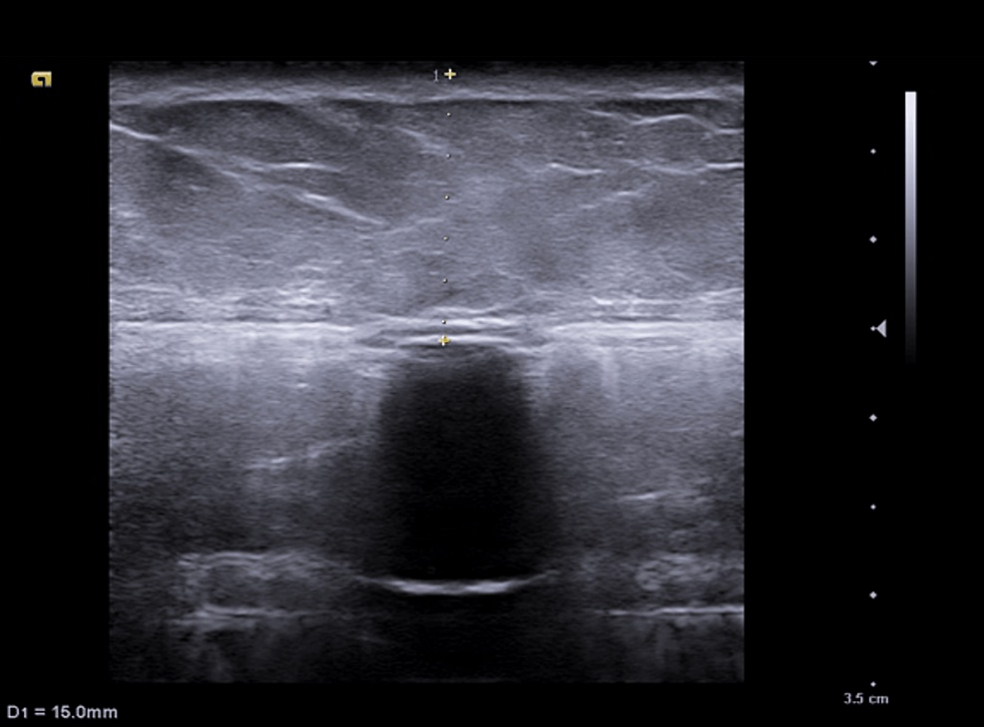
Ultrasound imaging of ITB pump. The surface of the intrathecal pump is identified by a hyperechoic line with acoustic shadowing. A hypoechoic shadow represents the RFP deep into the silicone membrane. Distance skin–pump entry (D1): 15 mm. ITB: intrathecal baclofen; RFP: reservoir fill port
